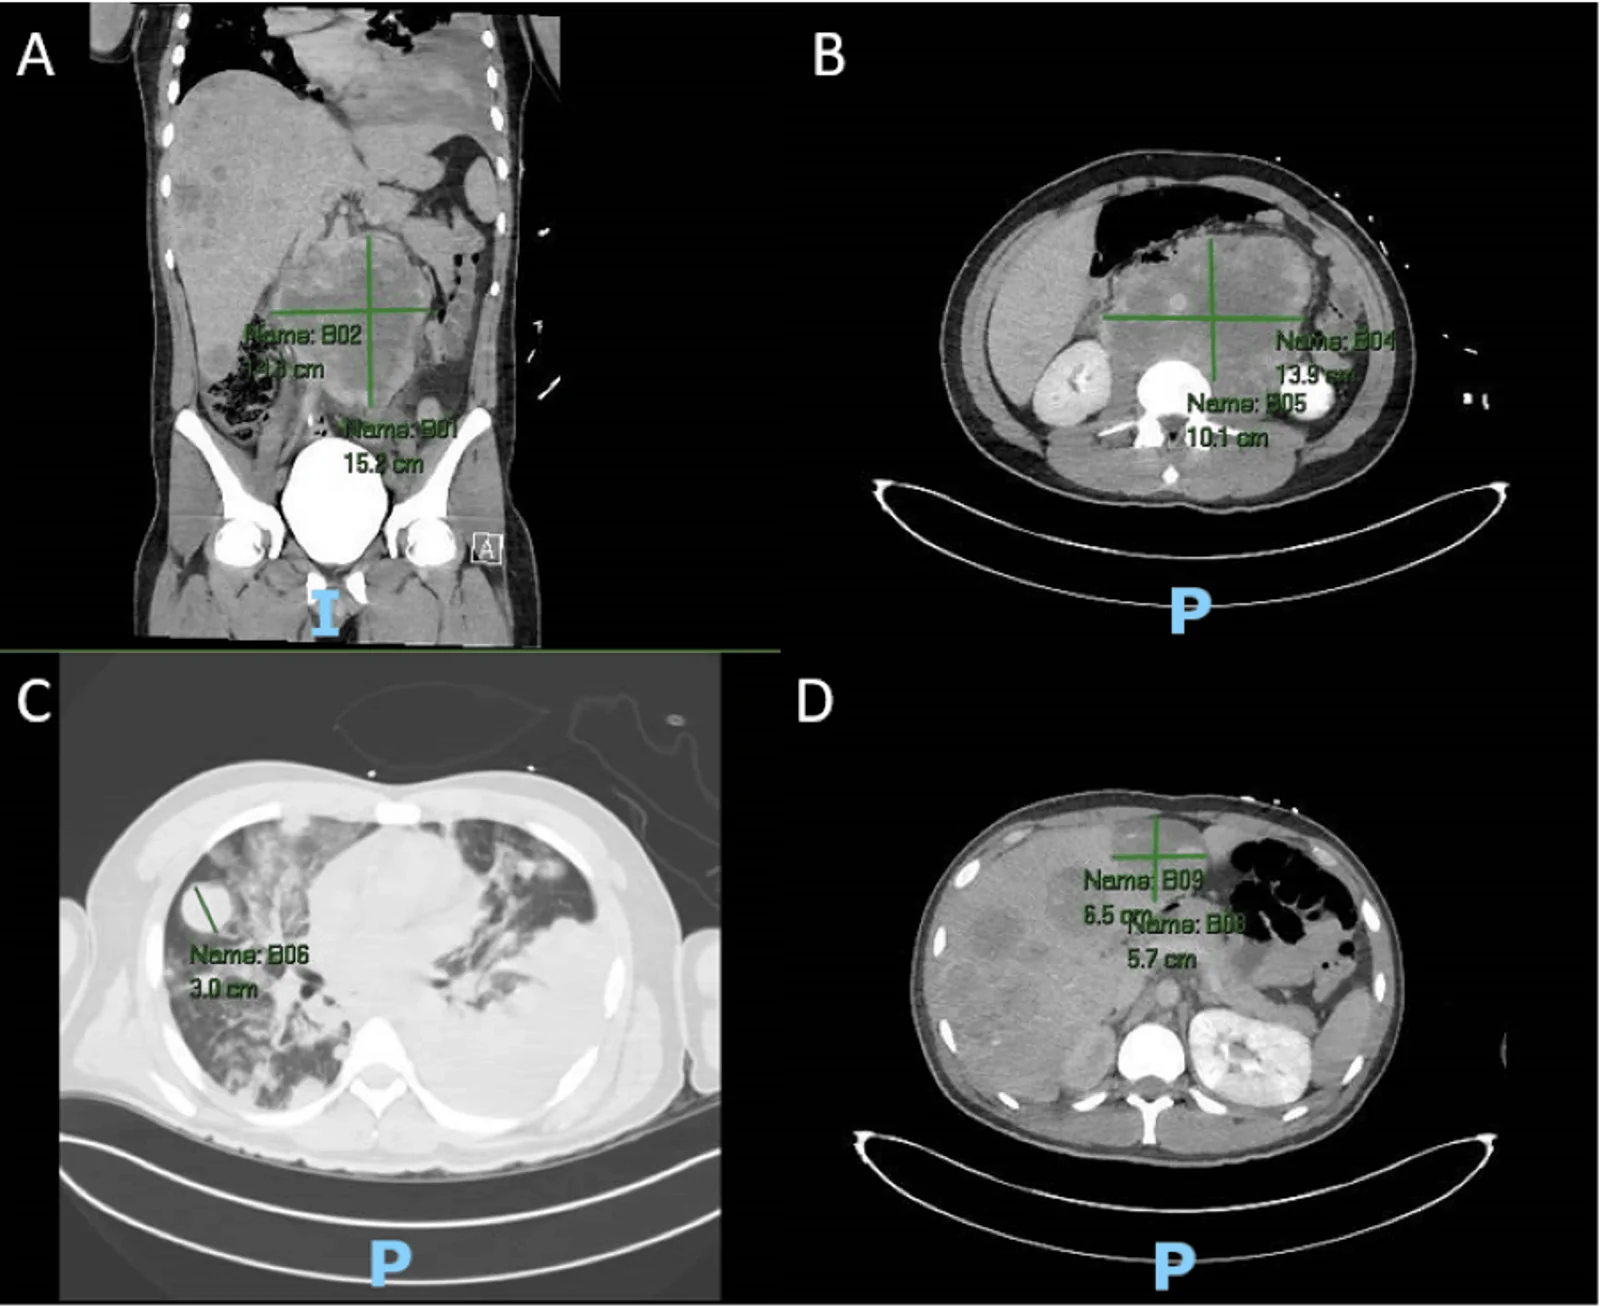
CT of the abdomen and pelvis with intravenous contrast. Contrast-enhanced computed tomography of the abdomen and pelvis, in coronal (A) and transverse (B) views, demonstrating a large retroperitoneal mass measuring approximately 14.3 cm x 15.2 cm and 10.1 cm x 13.9 cm, respectively, encasing the abdominal aorta (beginning just below the level of the superior mesenteric artery), bilateral renal arteries, inferior mesenteric artery, and left common iliac artery. The mass abuts and extrinsically compresses the inferior vena cava, left common iliac vein, and bilateral psoas muscles (left greater than right). Imaging also demonstrates numerous bilateral pulmonary nodules, at least 25, measuring up to 3 cm in size (C) and numerous hypoattenuating hepatic lesions, at least 20, with the largest being 6.5 cm x 5.7 cm (D).
